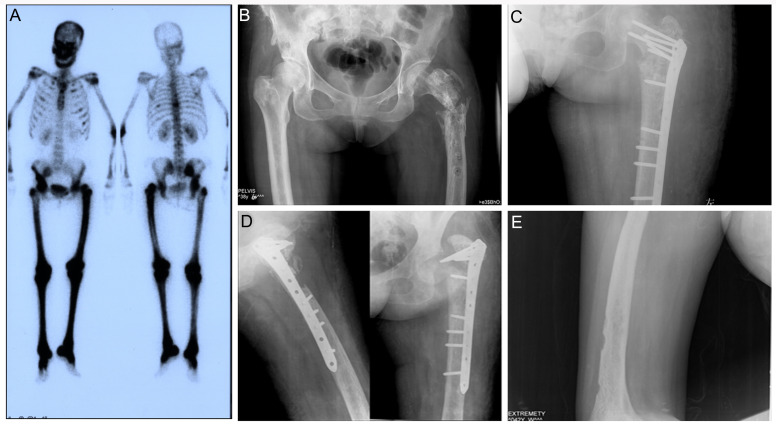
Patient 6: (A) ${}^{99m}$Tc-MDP bone scan showed increased uptake in the metaphyses and diaphyses of long bones, iliac bones and ribs. (B) Subsequent X-ray examination of pelvis revealed fracture near greater trochanter of the left femur. Postoperative X-ray examination (C) and X-ray examination performed 3 years after the internal fixation surgery (D) demonstrated disunion of the fractured femur. (E) X-ray of the left femur revealed diffuse osteosclerosis with focal osteolysis in the diaphysis and metaphysis of the distal right femur.
